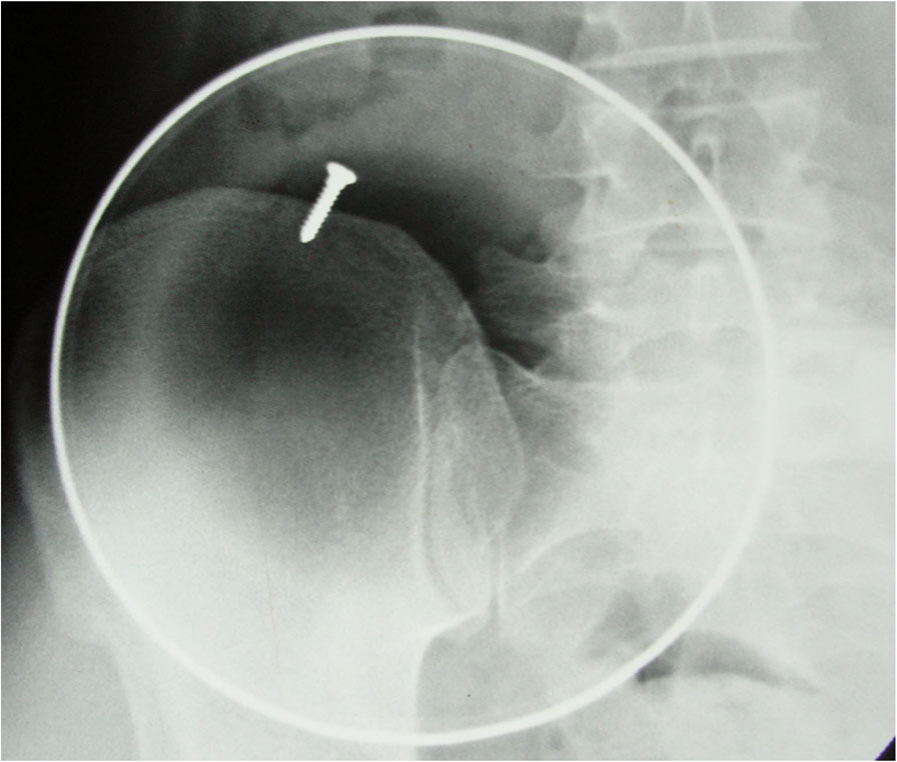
Radiographs showing the screw migrated in the colon
